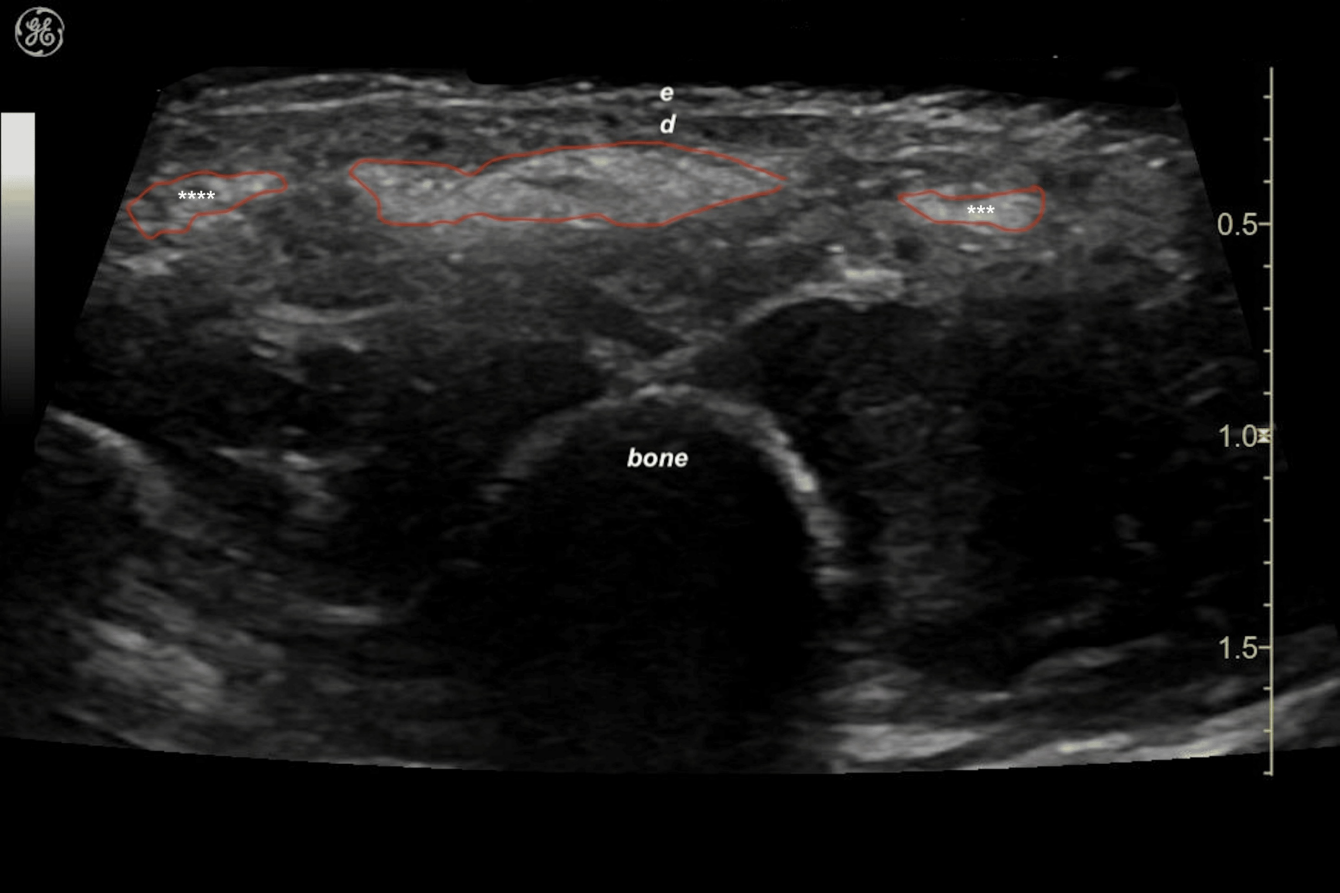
Sonographic appearance of factory-prepared CaHA–hyaluronic acid hybrid filler (HarmonyCa®) in the dorsal hand (transverse view) Red boundary lines outline hyperechoic deposits with echogenicity and band-like distribution similar to CaHA alone, but with more lobulated contours; isolated globular foci are marked by asterisks (*). Epidermis (e) and dermis (d) are labeled. Product shown: HarmonyCa® (Allergan Aesthetics, an AbbVie company, Irvine, CA, USA). Abbreviations: CaHA, calcium hydroxylapatite; HA, hyaluronic acid
